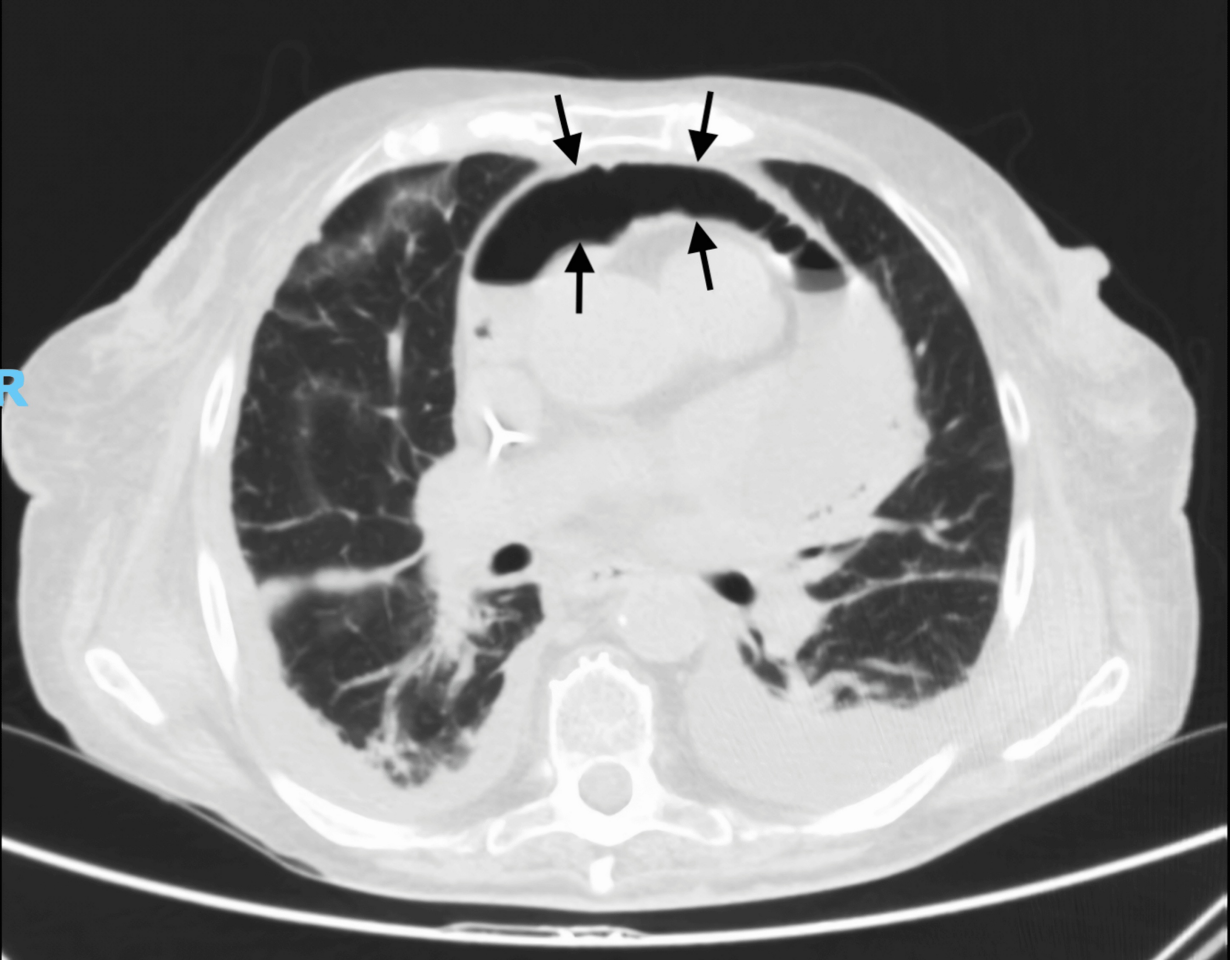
Axial contrast-enhanced CT of the chest in a pulmonary window setting (lung window). The image shows a large pericardial abscess with a distinct air–fluid level, causing partial compression of adjacent cardiac structures.
